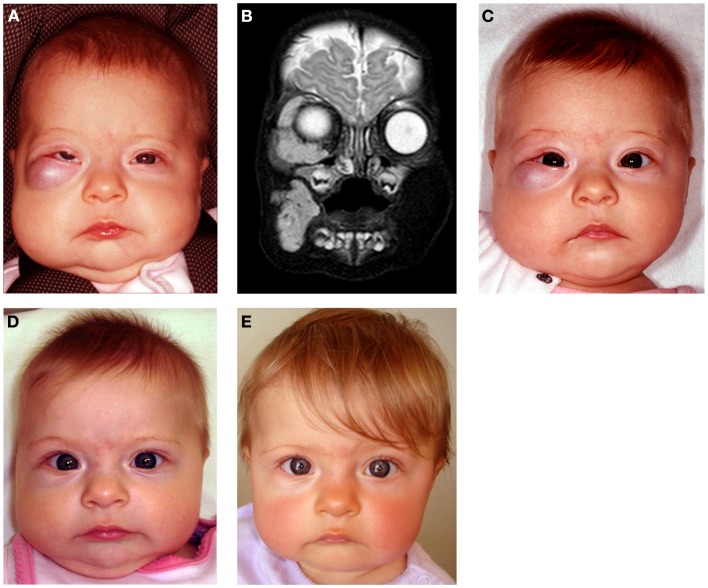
A 4-month-old girl presented with a rapidly growing infantile hemangioma on the right cheek, lower lid, and orbit with ocular dystopia (A) shown on a T2-weighted MRI scan (B). Accelerated involution of the lesion with equalization of the globe 7 days (C), 4 weeks (D), and 5 months (E) following institution of propranolol therapy at 2 mg/kg/day. Reproduced with permission from Plastic and Reconstructive Surgery (70).
